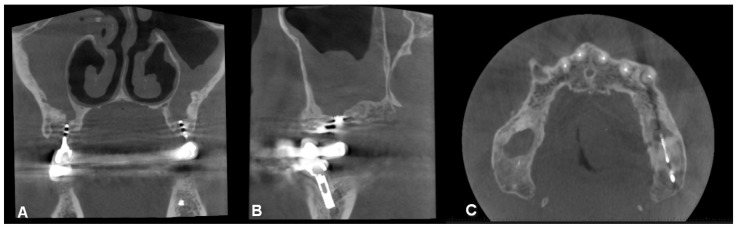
CBCT image of bilateral maxillary sinusitis caused by blade implants; chronic OAF (oroantral fistula) next to the implants. ((A): coronal, (B): sagittal, (C): axial view).
